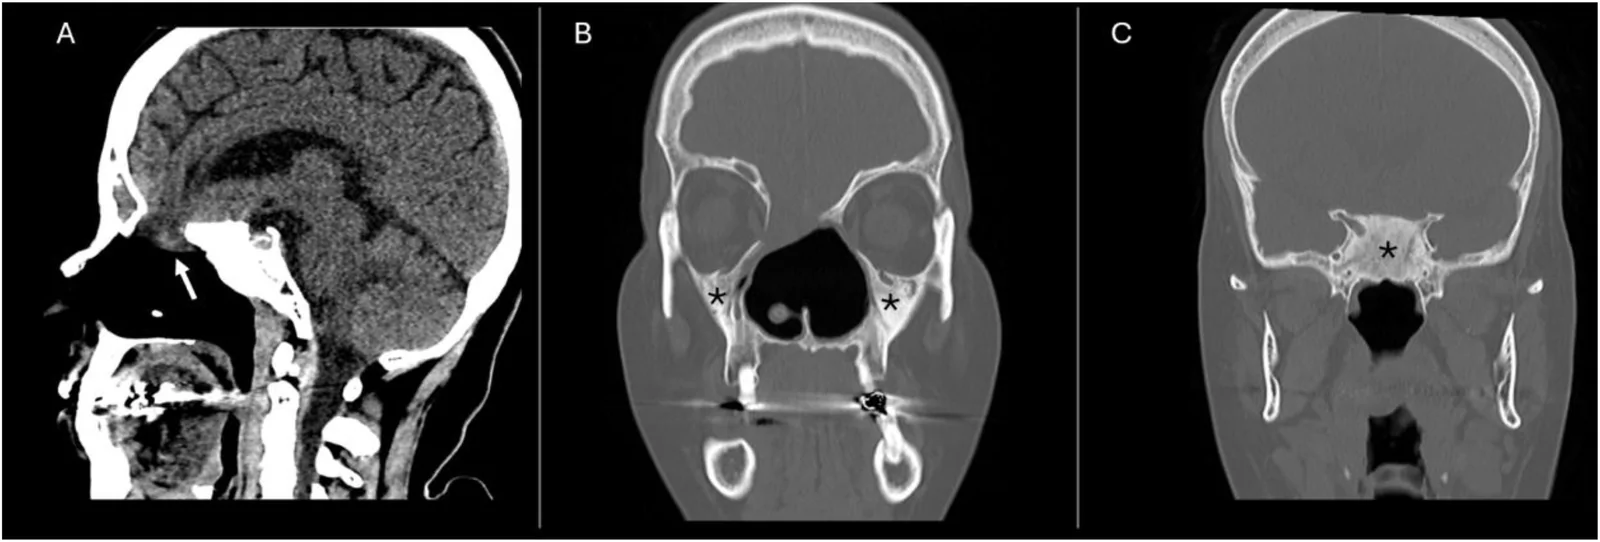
Computed tomography images of the paranasal sinuses. (A) Sagittal soft-tissue window image shows a right ethmoidal roof defect with herniation of brain parenchyma (arrow). (B) Coronal bone window image shows extensive osseous destruction of the nasal cavity and ethmoid air cells, with sclerotic changes in the maxillary sinuses (*). (C) Coronal bone window image shows sclerotic change in the sphenoid sinus (*).
